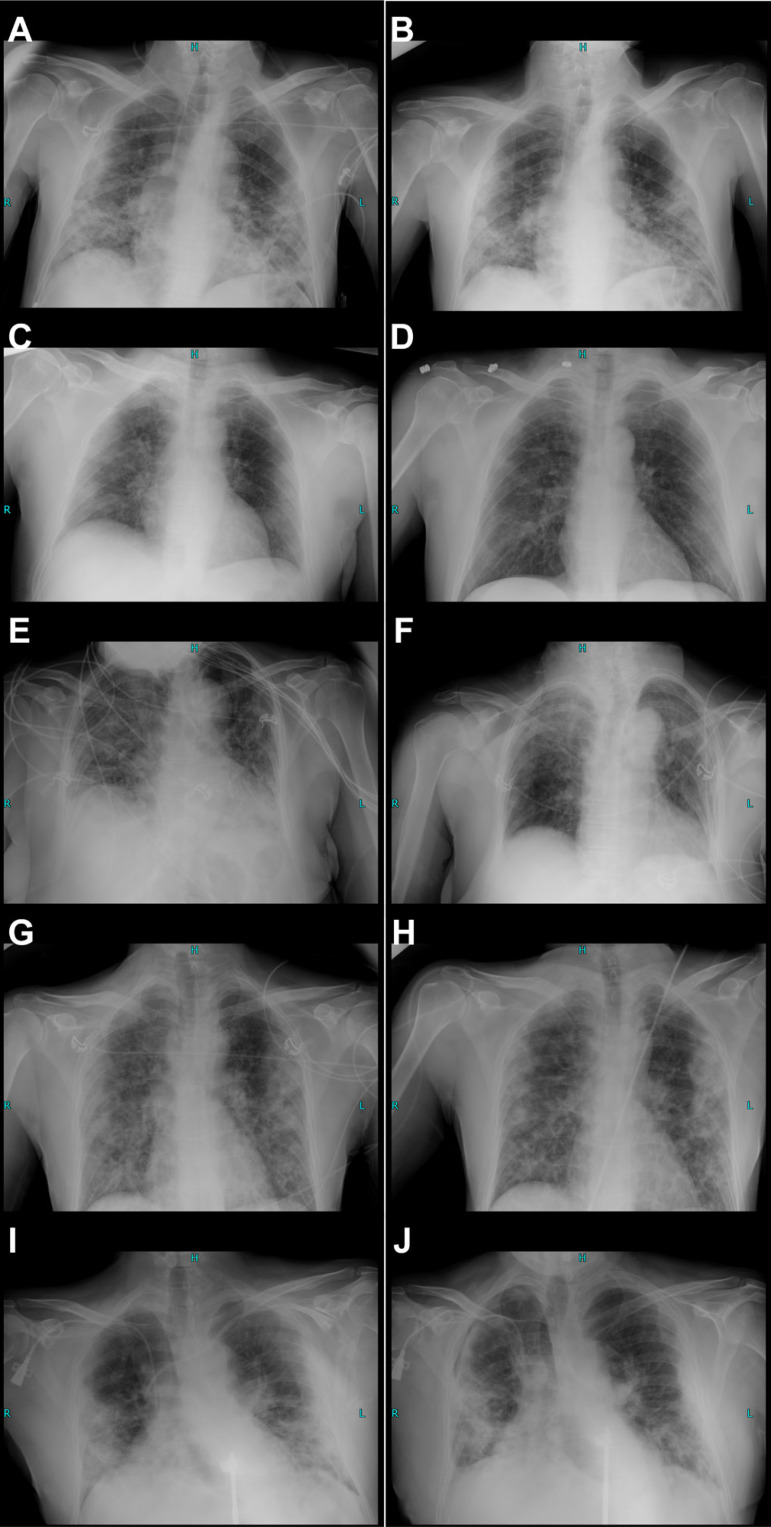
Pre- and post-treatment X-ray images. (A) 62-year-old male (Figure 6M) was admitted with oxygen
